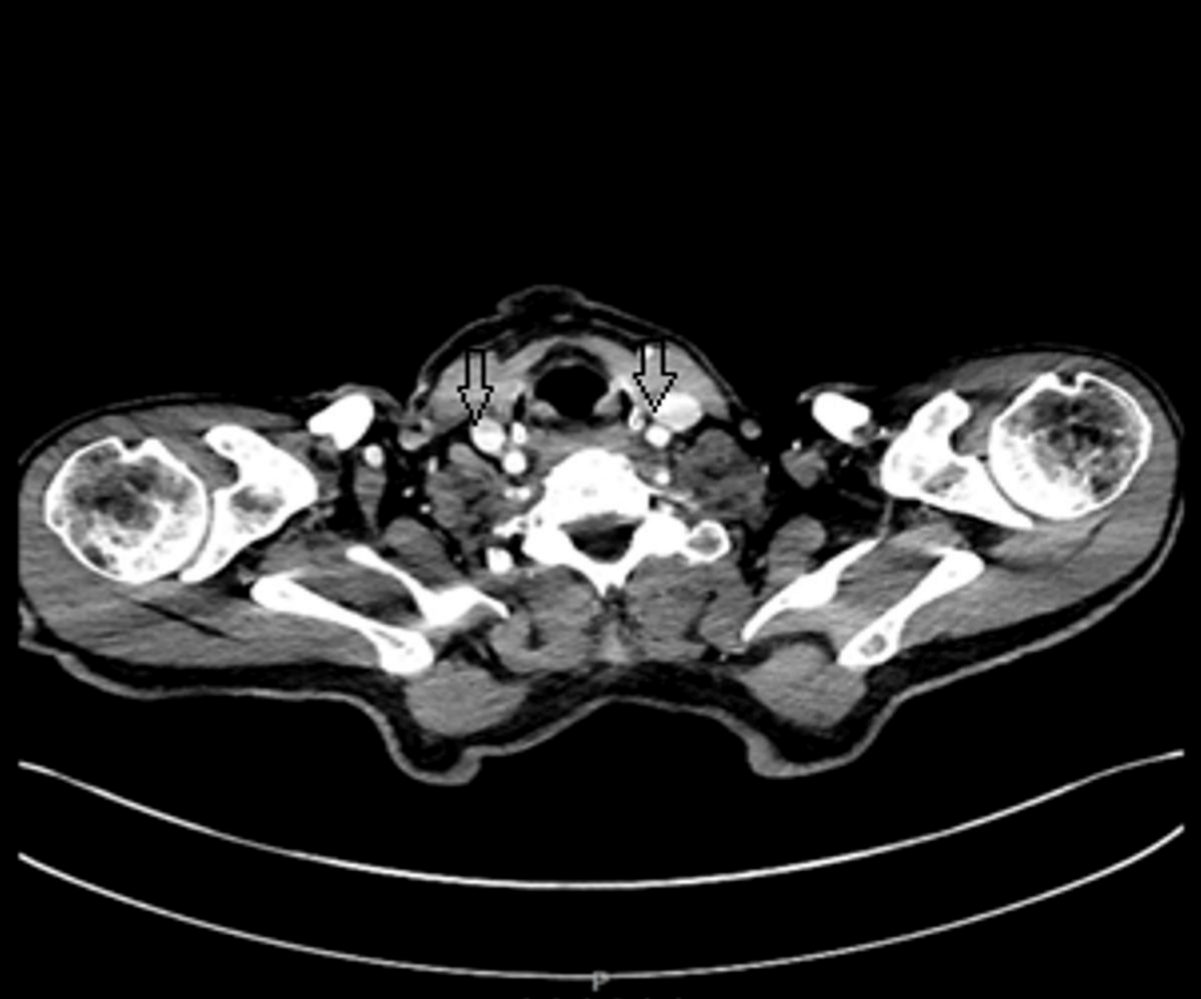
The CT scan of the neck with contrast revealed no evidence of internal jugular vein thrombus suggestive of Lemierre syndrome.
